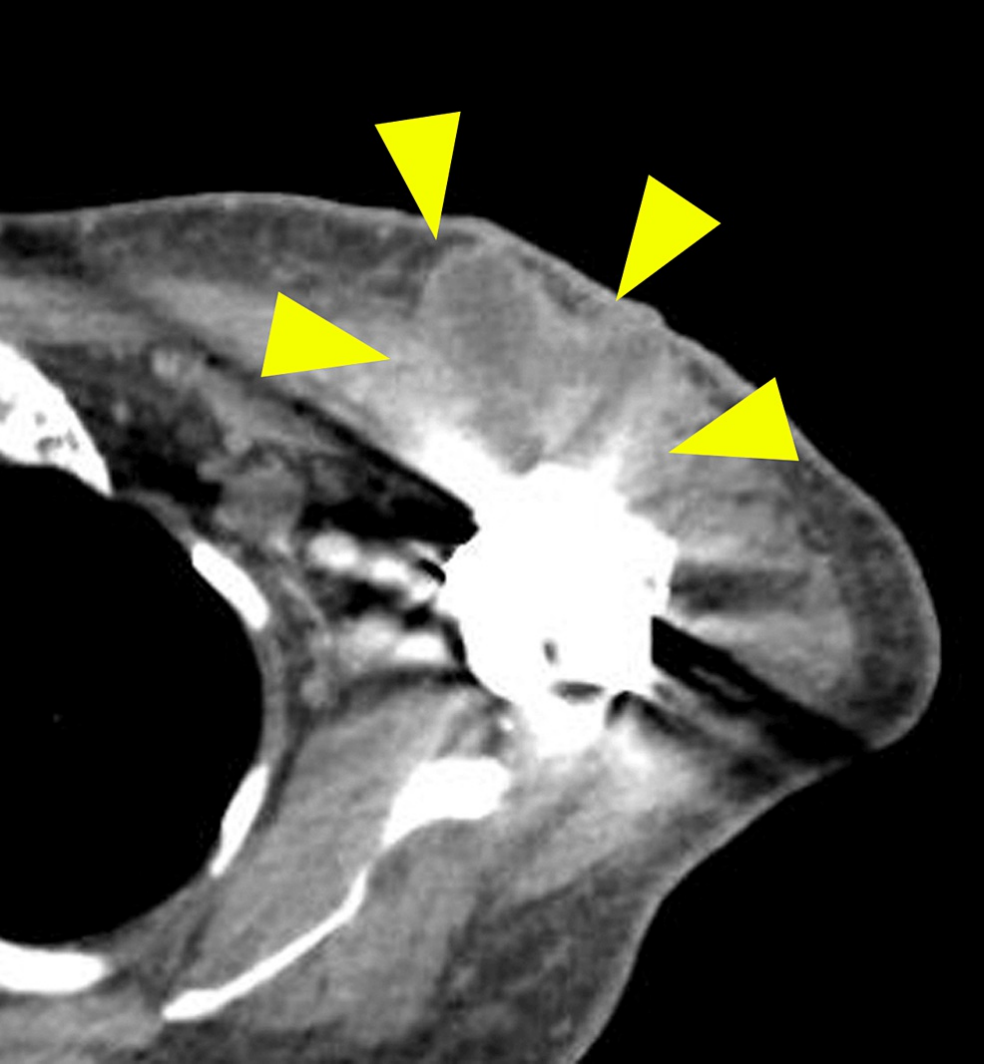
Enhanced computed tomography Yellow arrowheads indicate an infected lesion with rim enhancement spreading subcutaneously to the periprosthetic space.
